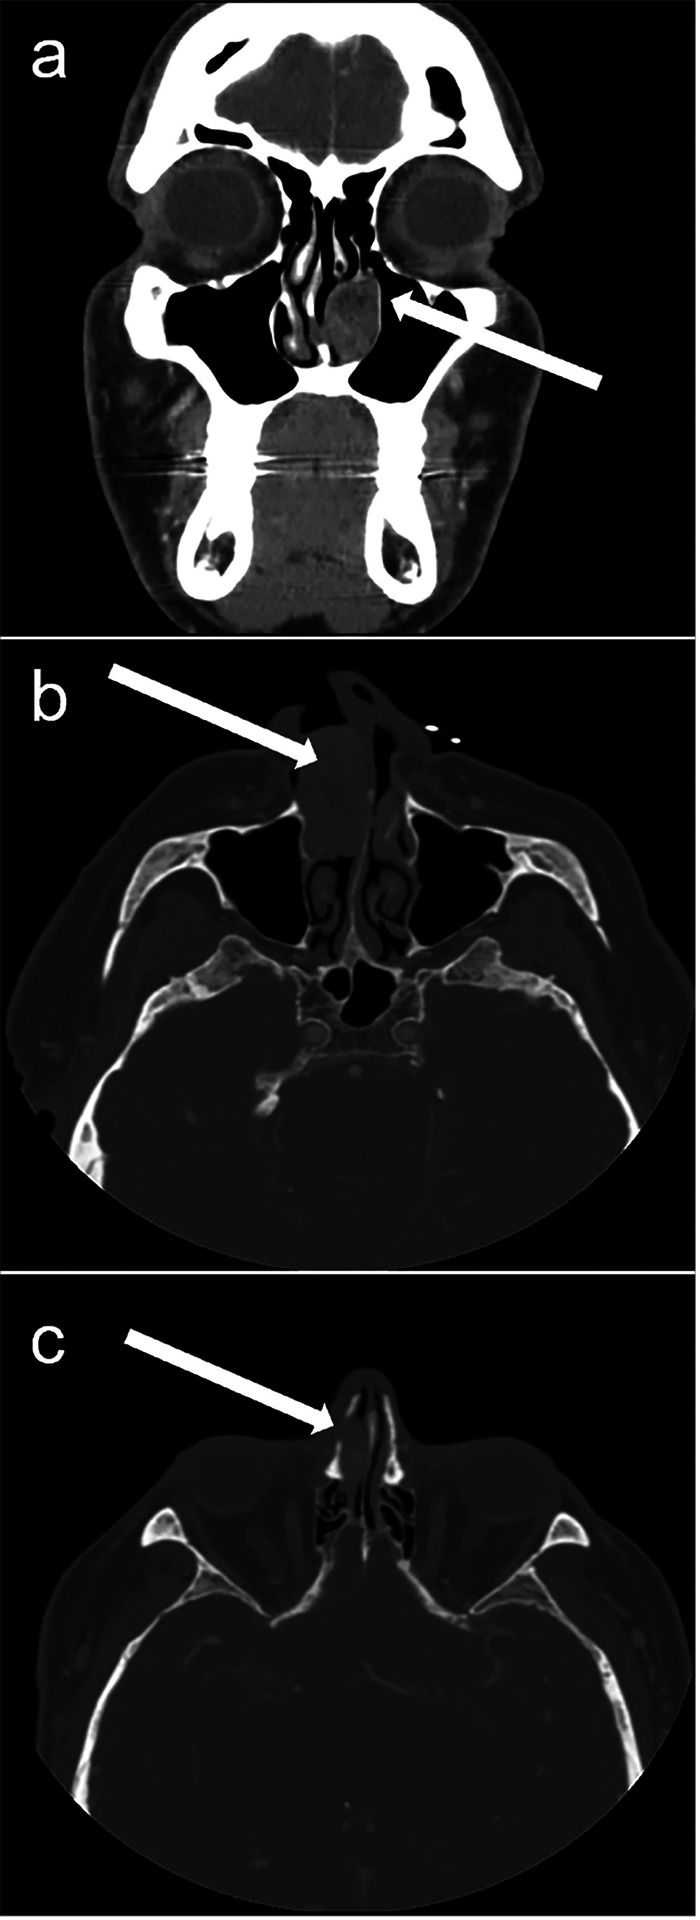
Radiographic features of nasal cavity angiomyolipomatous lesions. Computed tomography images portraying, by white arrows, a left nasal lacrimal duct mass with osseous remodeling (patient #2) (a) and a right nasal mass (b) with new sites of osseous erosion (c) (patient #1)
